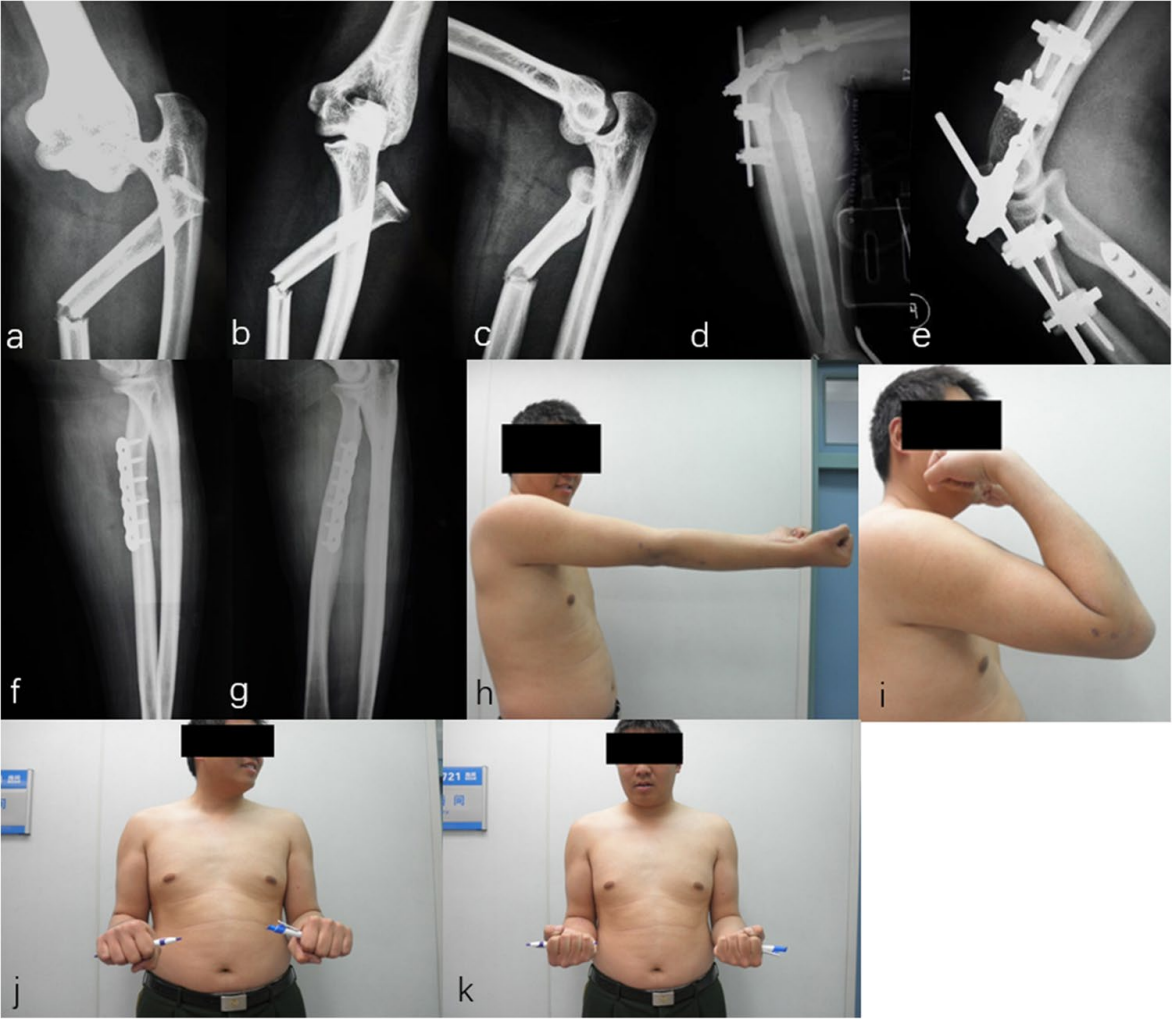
Radiographs of a 44-year-old right-hand dominant male exhibiting a DDE and a radial shaft fracture (a–c). Post-operative radiographs of the elbow showed good reduction and fixation with an external fixator and an ORIF of the radial shaft (d–e). The external fixator was removed at 6 weeks post-operative (f–g) and he has full range of motion at 18 monthly follow-up (h–k)
